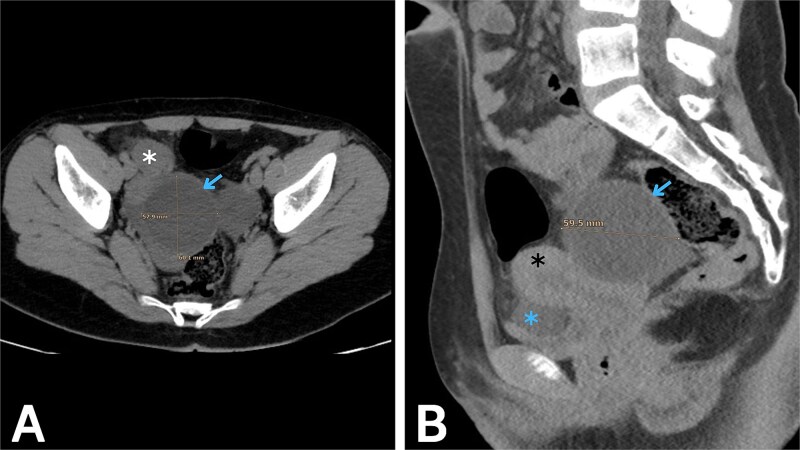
Abdominopelvic CT without contrast. (A) Axial and (B) sagittal images show a right paraovarian cystic lesion (blue arrow) measuring approximately 6 × 5.8 cm, associated with a small volume of pelvic free fluid (blue asterisk). The right ovary (white asterisk) and the uterus (black asterisk) are also seen.
